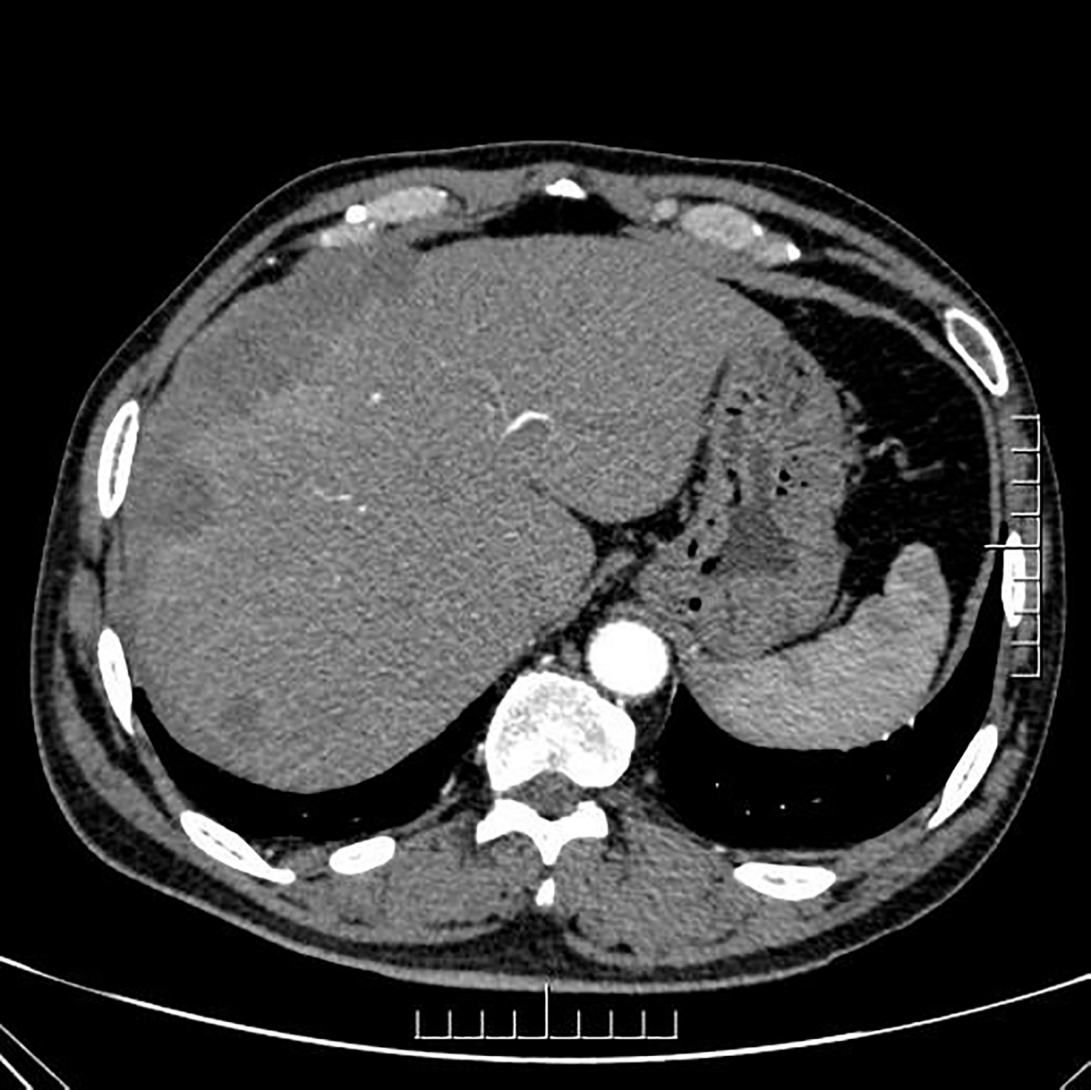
Axial contrast-enhanced CT image of the abdomen during the late arterial phase showing multiple nodular and sheet-like low-attenuation lesions with peripheral enhancement in the right subdiaphragm and right anterior and left medial lobe of the liver.
